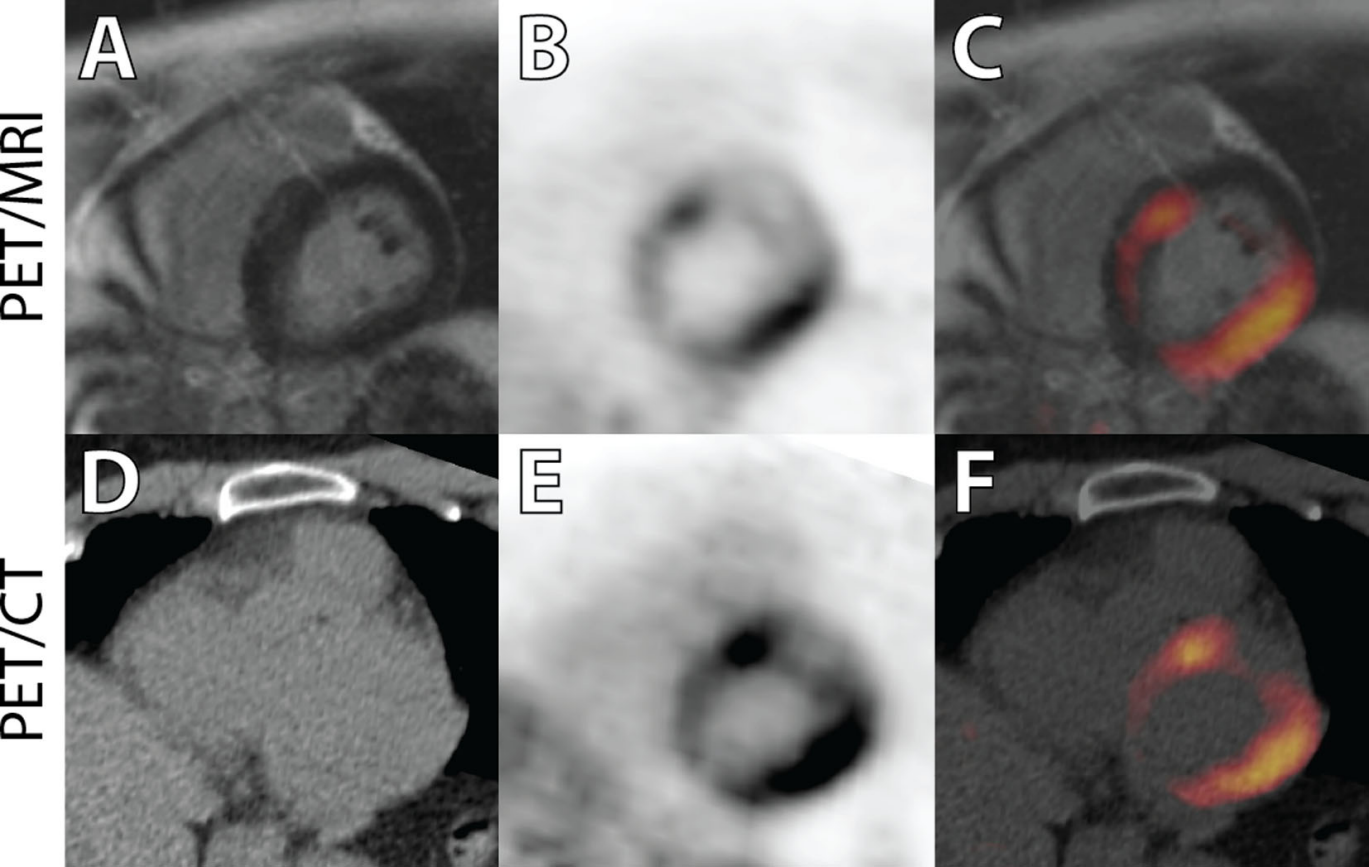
Images presented from patient 7 in a similar format as for Fig. 2. In this image, there is no area of signal enhancement on the delayed enhancement MR image (A). However, there is a marked increase in uptake, essentially transmural in the lateral wall and anterior septum (B, C). There is somewhat greater definition as to the extent of this uptake seen on the PET/MR images vs the PET/CT images (E, F). This patient’s ejection fraction was 61% with moderate septal hypokinesis
